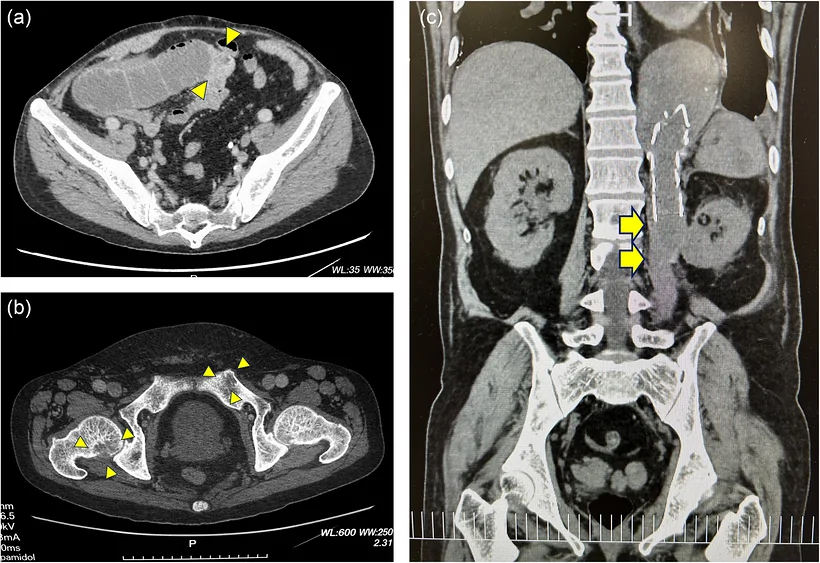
Computed tomography (CT) scan. (a) Contrast‐enhanced CT showing focal jejunal wall thickening with associated luminal stenosis at the bleeding site (arrowheads). (b) CT bone window demonstrating multiple suspected bone metastases (arrowheads). (c) Abdominal aortic graft with soft‐tissue infiltration (arrows).
